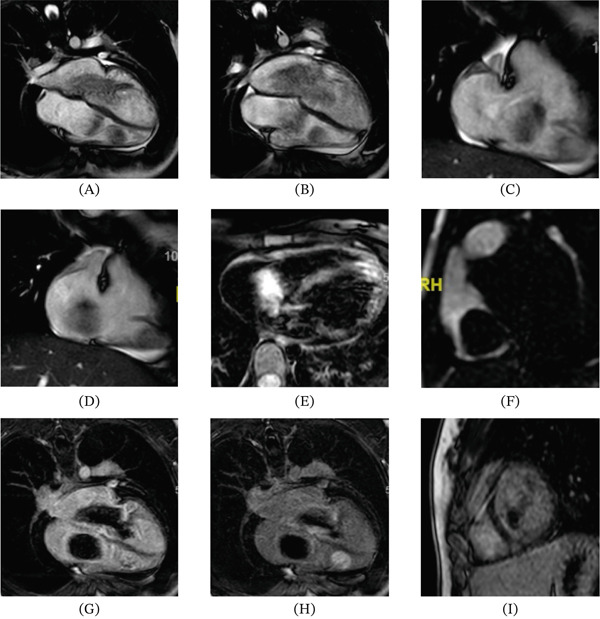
Cardiac magnetic resonance imaging demonstrating multiple intracardiac masses: (A, B) four‐chamber long‐axis cine images showing mobile right and left atrial masses with protrusion through the atrioventricular valves. (C, D) Right ventricular long‐axis cine images demonstrating a third mass attached to the mid–apical free wall of the right ventricle. (E) Fat‐suppressed T2‐weighted sequence showing hyperintensity of the masses. (F) First‐pass perfusion image showing no contrast uptake by the masses. (G) Early gadolinium enhancement image demonstrating peripheral enhancement of the left atrial tumor. (H) Late gadolinium enhancement atrial‐level image showing enhancement of the left atrial tumor, with no enhancement of the right atrial tumor. (I) Late gadolinium enhancement image demonstrating enhancement of the right ventricular tumor.
